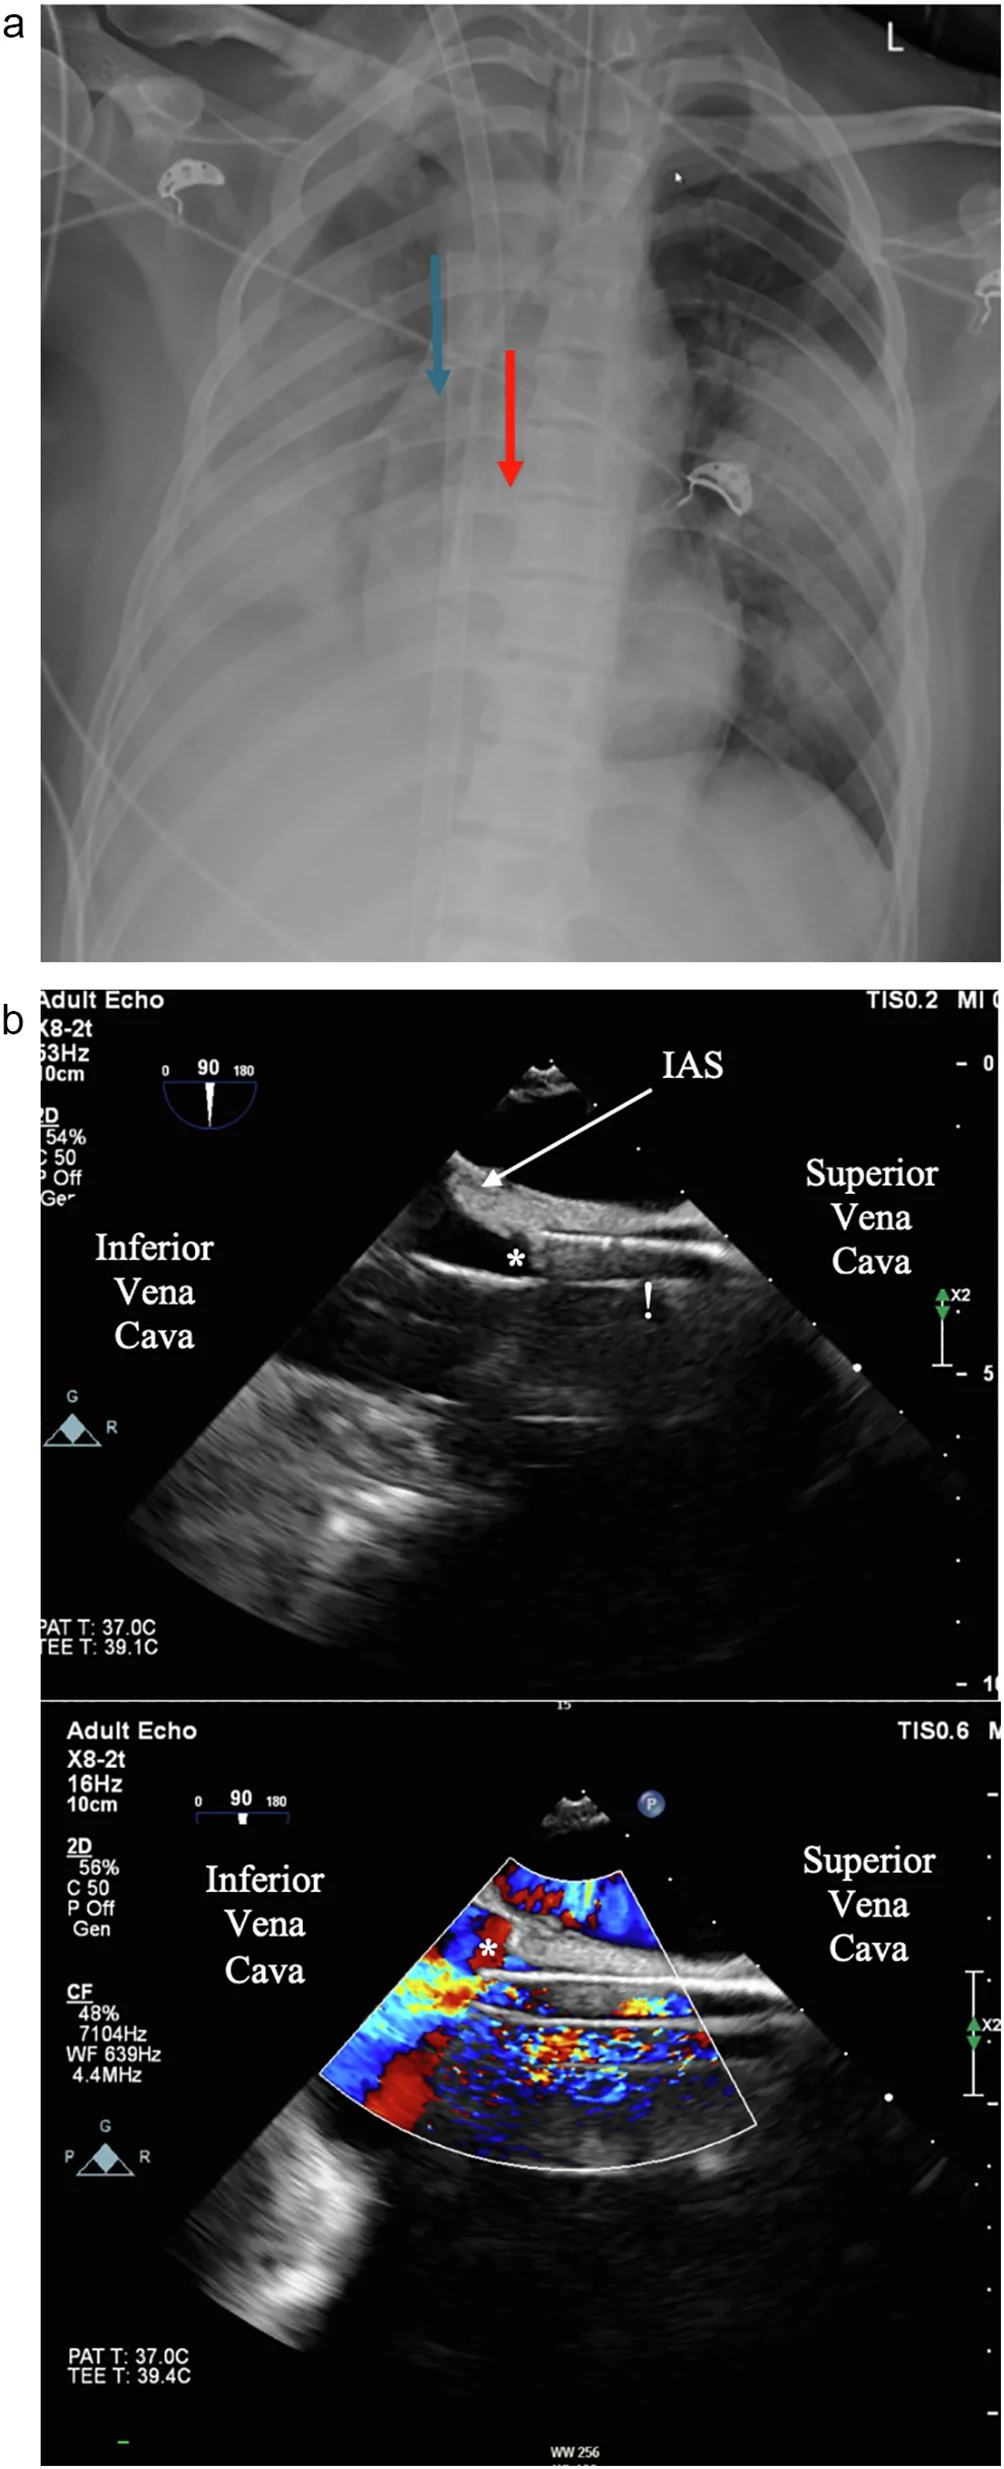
Plain film radiograph demonstrating a femoral multistage drainage cannula with tip in the SVC, and a right internal jugular vein single-stage return cannula with tip in the right atrium. Overlap and direction of inflow and return annotated with arrows: blue indicates drainage flow, and red indicates return flow. Key: SVC, Superior Vena Cava.
